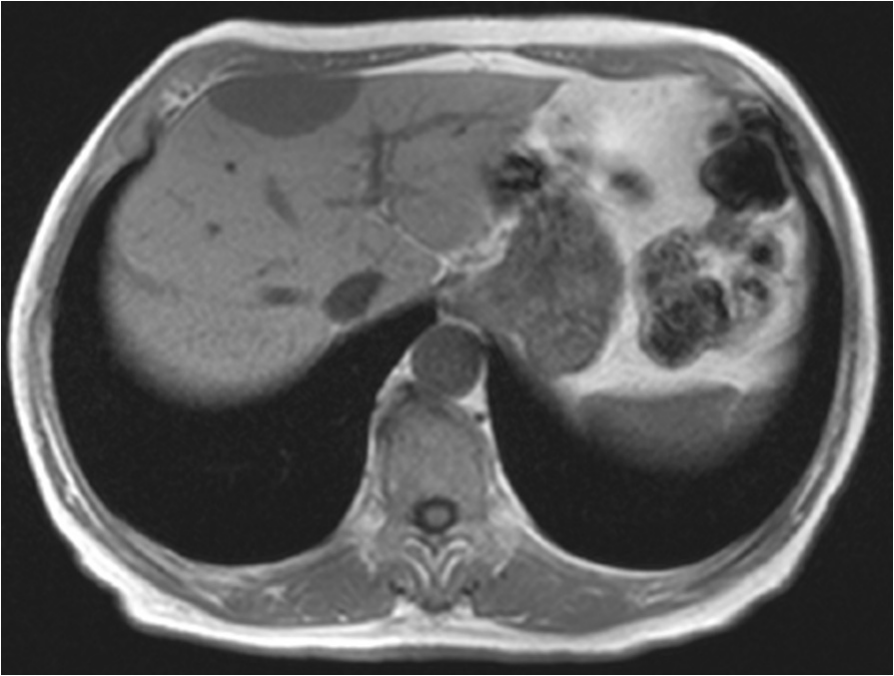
Axial in-phase gradient echo (GRE) T1-weighted magnetic resonance imaging (MRI) scan, obtained as a part of dual GRE (chemical shift) sequences, showing a focal mass in the right lobe to be hypointense when compared to liver parenchyma, but iso-intense compared to spleen parenchyma.
